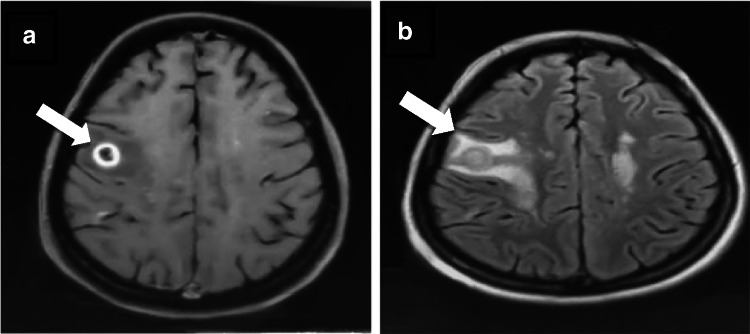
a Axial post-contrast T1W images showing ring enhancing lesion in the right parieto-frontal region. b Axial post-contrast T2/FLAIR images showing perilesional edematous change around the ring enhancing lesion
